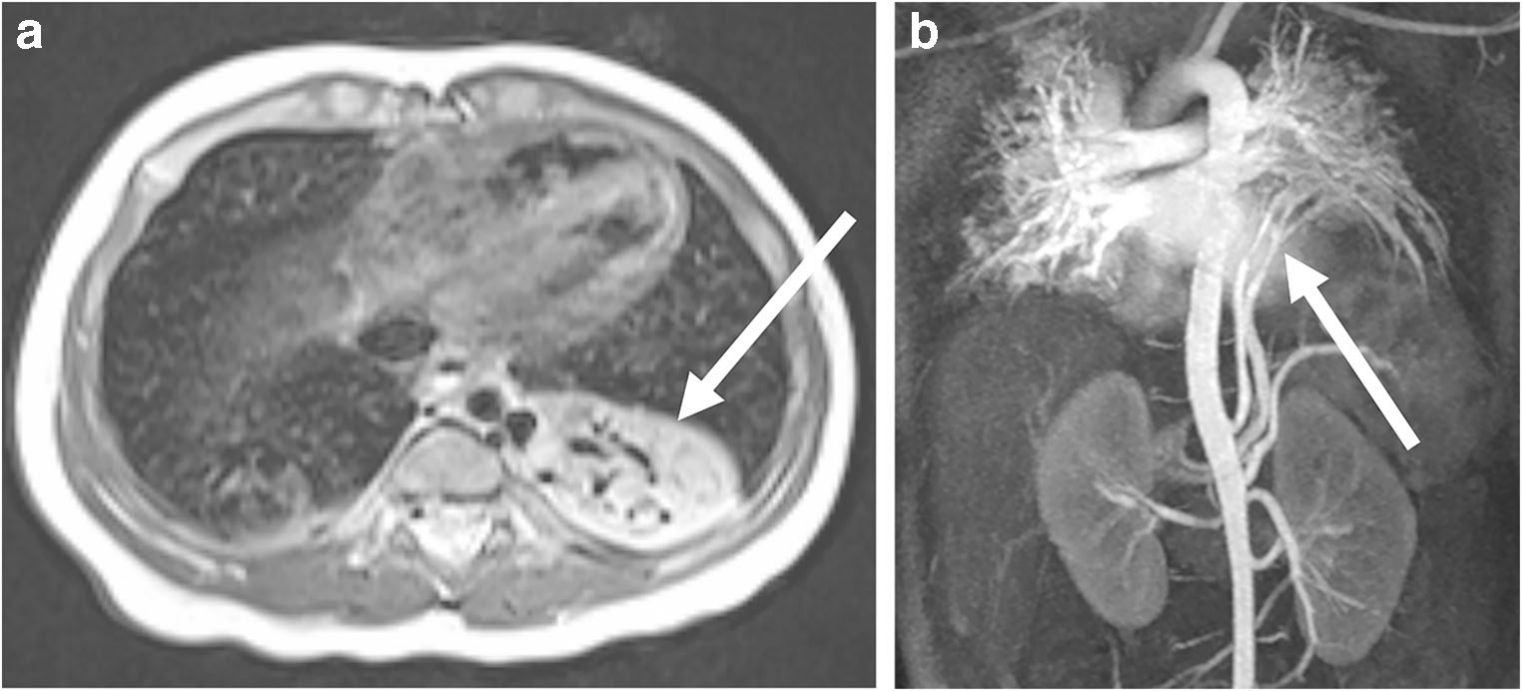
Pulmonary sequestration on lung MR in a 6-month-old boy. a Axial T2-weighted turbo spin-echo MR image (parameters in Table 2) identifies the supradiaphragmal lesion on the left (*arrow*) as a pulmonary sequestration by the low-signal vessels. b Coronal MR angiography proves the diagnosis by demonstrating the atypical arterial supply and venous drainage (*arrow*)
